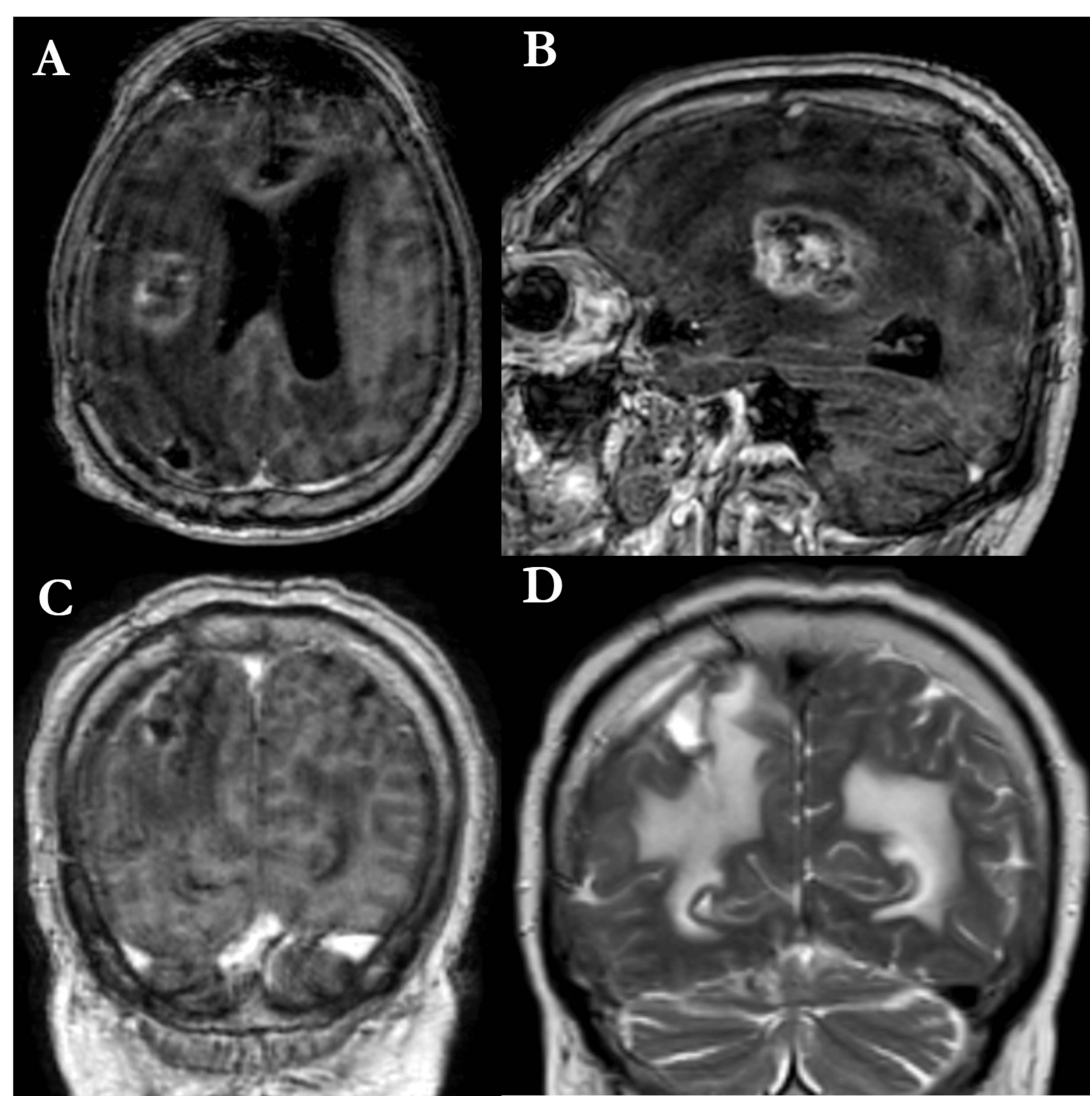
Three months postoperative and post-radiation therapy brain contrast-enhanced MRI (A) Axial T1-weighted contrast-enhanced sequence. (B) Right paramedian sagittal T1-weighted contrast-enhanced sequence. (C) Frontal T1-weighted contrast-enhanced sequence. (D) Frontal T2-weighted FLAIR sequence. The quality of the examination was affected by the patient’s agitated state. The post-radiation therapy brain MRI revealed that the insular lesion had increased in size, and peripheral contrast enhancement in the surgical bed was observed, indicating either radionecrosis or a relapse of the previously removed lesion.
